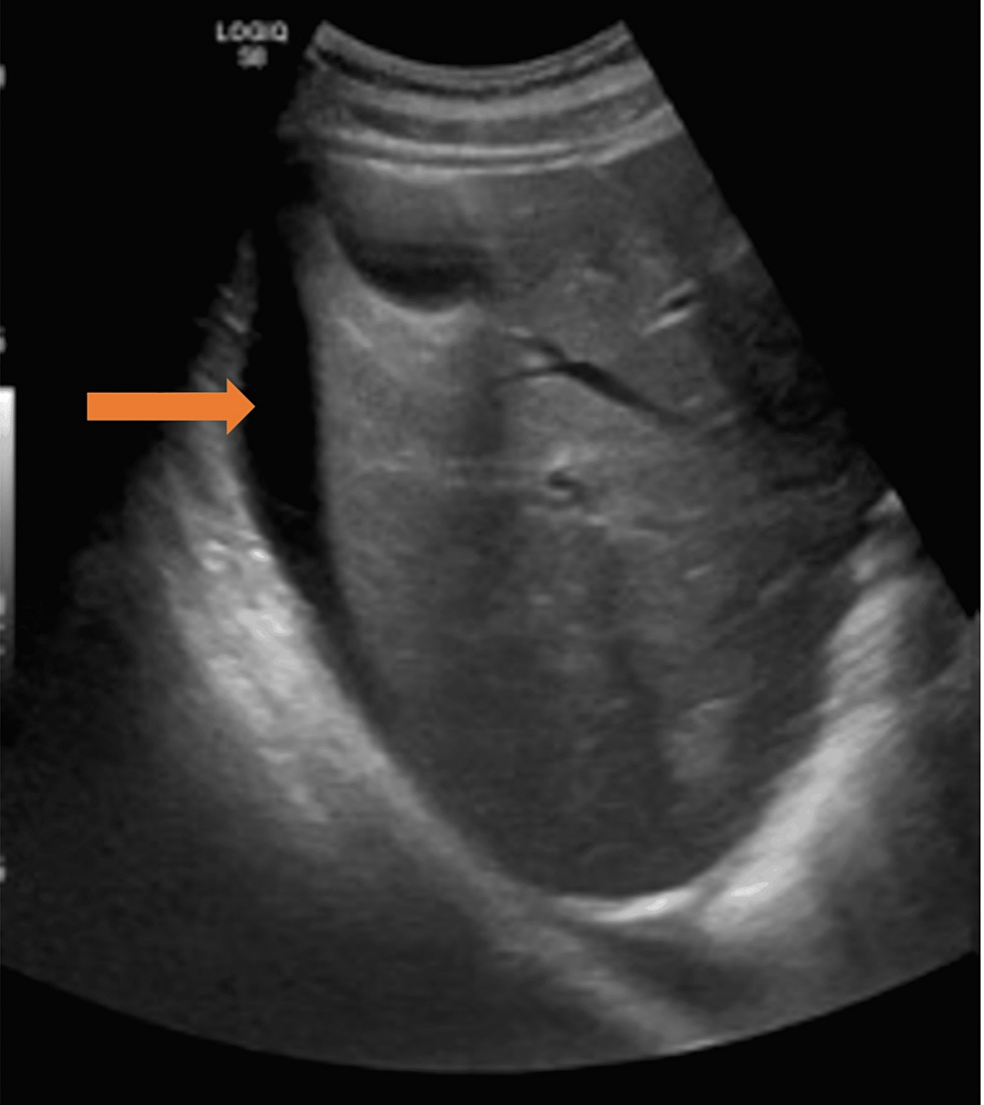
Abdominal ultrasound (orange arrow) highlighting moderate ascites in the right upper abdominal quadrant.
